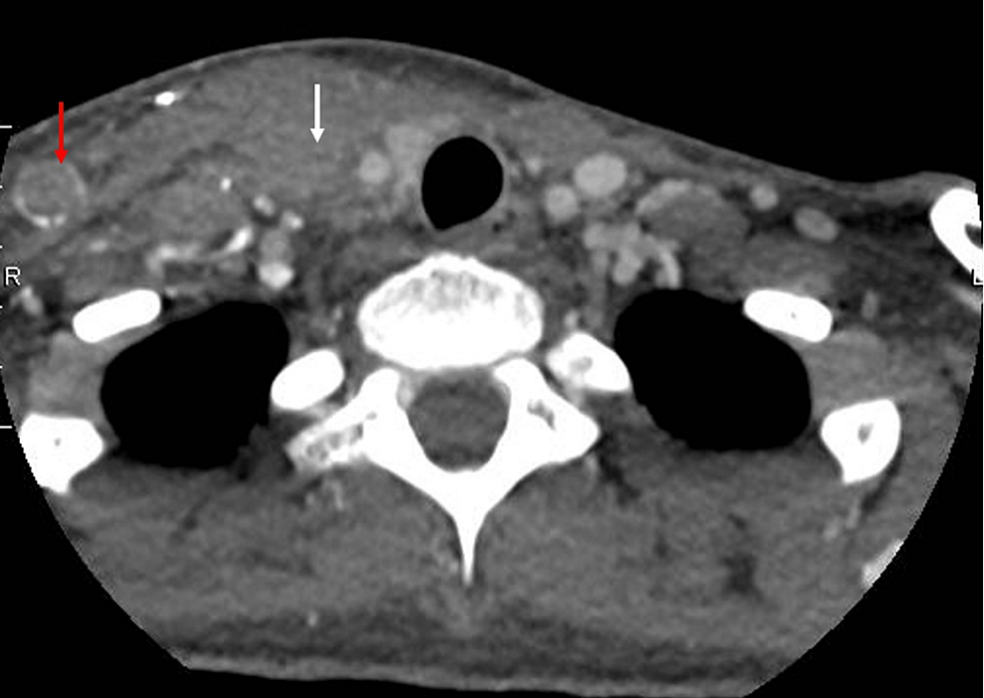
Computed tomography scan of head and neck demonstrating occlusion of the right internal (white arrow) and external (red arrow) jugular veins.
